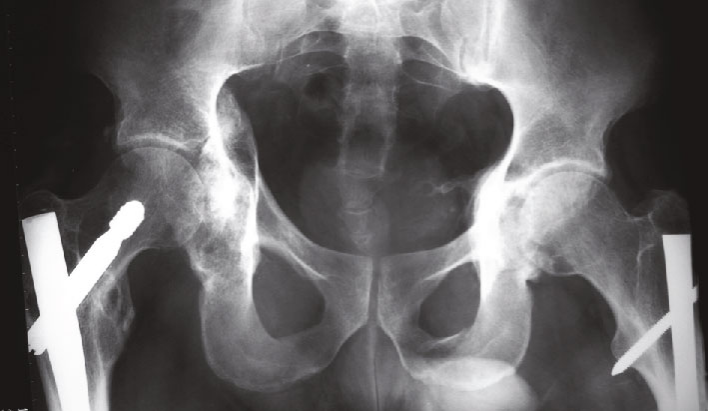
Follow-up X-ray at 10 months postsurgery.
